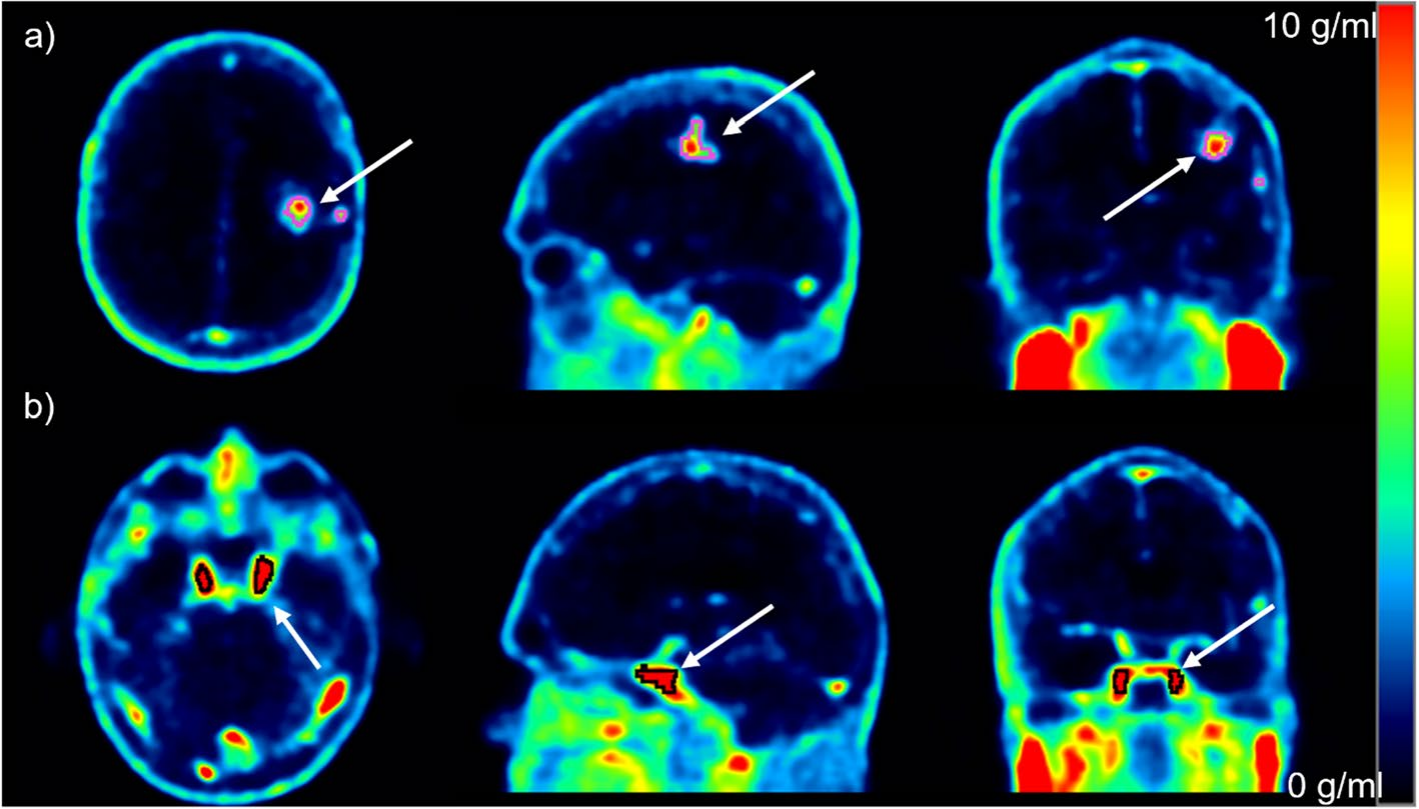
Standardized uptake value (SUV) image of a representative male adult patient with recurrent glioblastoma scanned with ^18^F-PSMA-1007. ^18^F-PSMA-1007 uptake and delineated VOIs are shown for tumour tissue (white arrow in a) and blood pool (white arrow in b)
